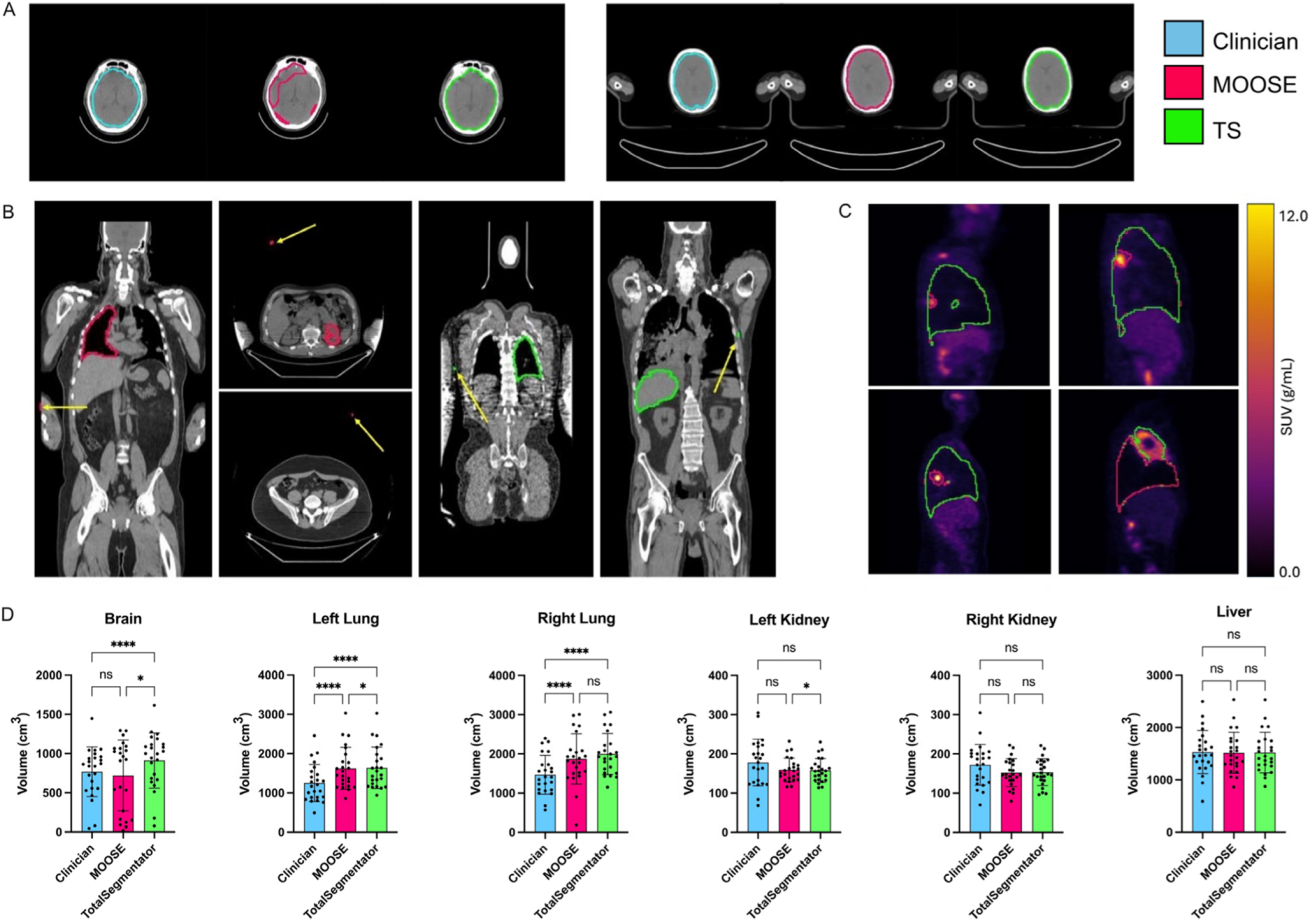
[^18^F]FDG-PET and CT delineation examples with volumetric comparisons between clinician, MOOSE and totalsegmentator generated VOIs: A: Examples where MOOSE struggled/did not struggle to capture the full brain VOI. B: Examples of false positive predictions far from the target VOI. Arrows point to false positive prediction. C: MOOSE was able to capture tumors present within the right lung, avoiding inclusion in the VOI. D: Volumetric comparisons between delineation methodologies. Significance Testing: One Way ANOVA.  indicates *p <* 0.0001, * indicates *p <* 0.05. MOOSE vs. TS Brain: *p* = 0.013, MOOSE vs. TS Left Lung: *p* = 0.043, MOOSE vs. TS Left Kidney: *p* = 0.049
